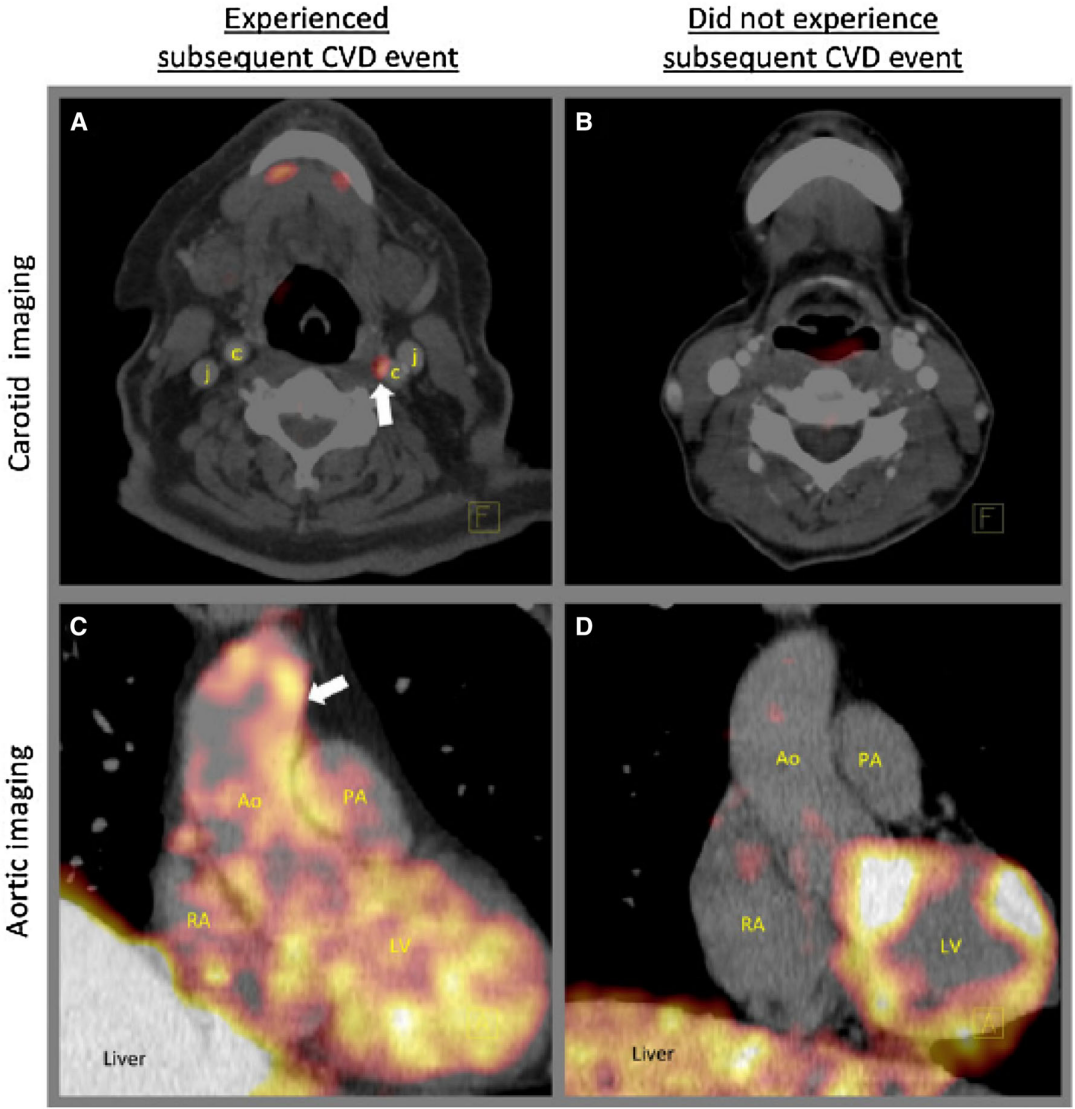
Example positron emission tomography images demonstrating high and low arterial ^18^F-fluorodeoxyglucose uptake in two individuals. One individual, who experienced a subsequent CVD event after imaging, (A and C), demonstrated high carotid FDG uptake (*white arrow*, A) and high aortic FDG uptake (*white arrow*, C) prior to that event. In contrast, a second individual who did not experience a subsequent, (B and D) manifested low arterial FDG uptake. Reprinted with permission^1^
